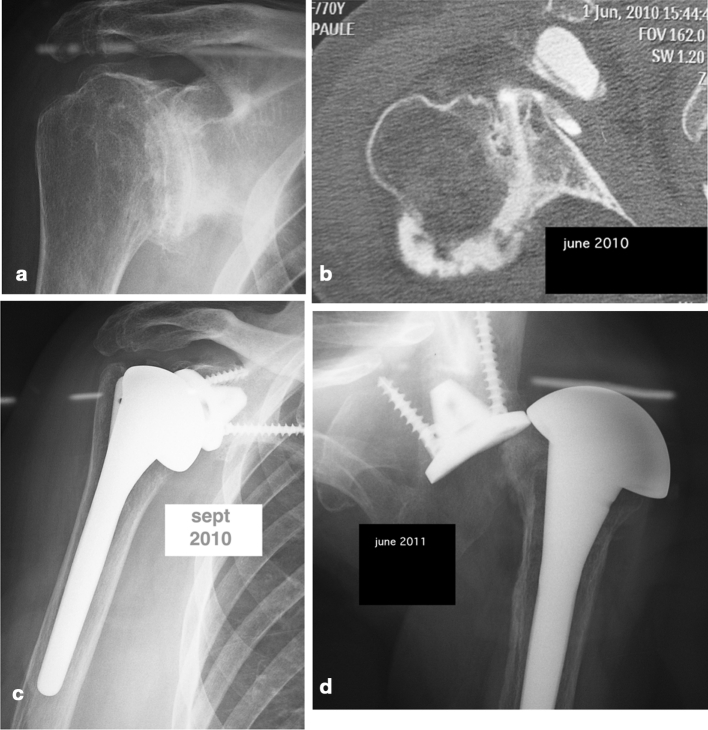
MB glenoid implanted on a type b2. A dislocation occured at 6 months. Conversion for a reverse shoulder arthroplasty: a preoperative X-ray, b preoperative CT scan showing the posterior subluxation, c immediate postop X-ray, d posterior dislocation at 6 monthsTwo secondary rotator cuff tears were converted to a reverse. The first one, at 17 months of the revision, had no pain but a fair active and passive mobility with a Constant score of 44 (68 %). The other one was a b2 glenoid and had also a contralateral MB glenoid with an excellent result. During revision performed at 2 years a posterosuperior, PE wear was found with a contact between the metal tray and the humeral head. At 36 months of the revision, the result was excellent with a Constant score of 64 (100 %), an active elevation of 140° (Fig. 3).
